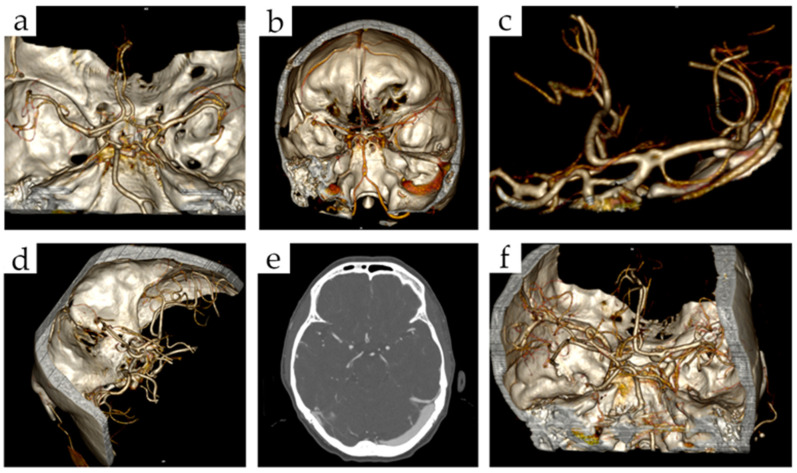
Cerebral arterial fenestrations on CT-3D reconstructions: (a) right ACA fenestration, (b) BA fenestration, (c) left MCA fenestration, (d) AComA fenestration, (e) AComA fenestration on CT angiography, (f) right MCA fenestration. (Abbreviations: ACA—anterior cerebral artery, BA—basilar artery, MCA—middle cerebral artery, AComA—anterior communicating artery).
